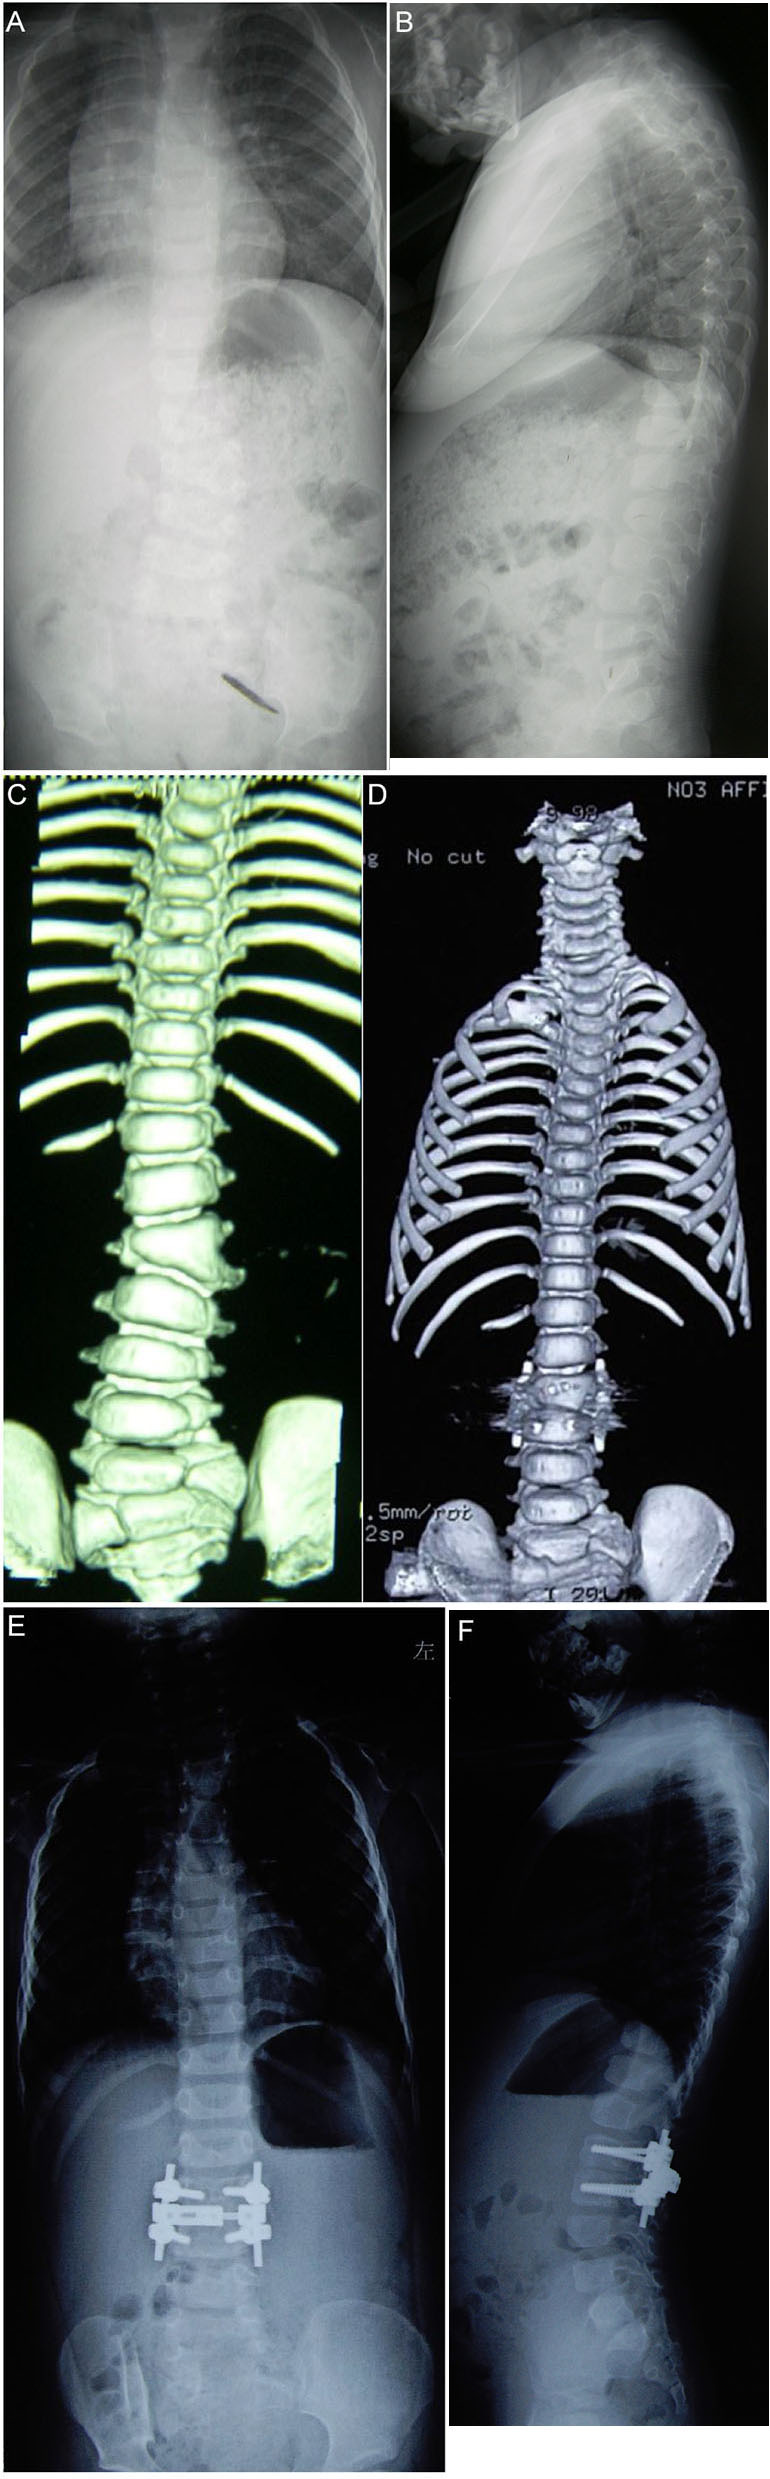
The child is a 2-year-old boy. Preoperative x-ray [(A) coronal position, (B) sagittal position]and computed tomography (CT) (C) reveal that local deformity left of the L3 hemivertebra is obvious, and hemivertebra resection *via* the posterior approach assisted with single segment bilateral pedicle screw internal fixation is performed. Postoperative CT (D) reveals that the osteotomy space is stable and the orthopedic effect is good. An x-ray 2 years after the operation revealed that the orthopedic effect was good, and there was no significant loss during follow-up ([(E) coronal position, (F) sagittal position].
